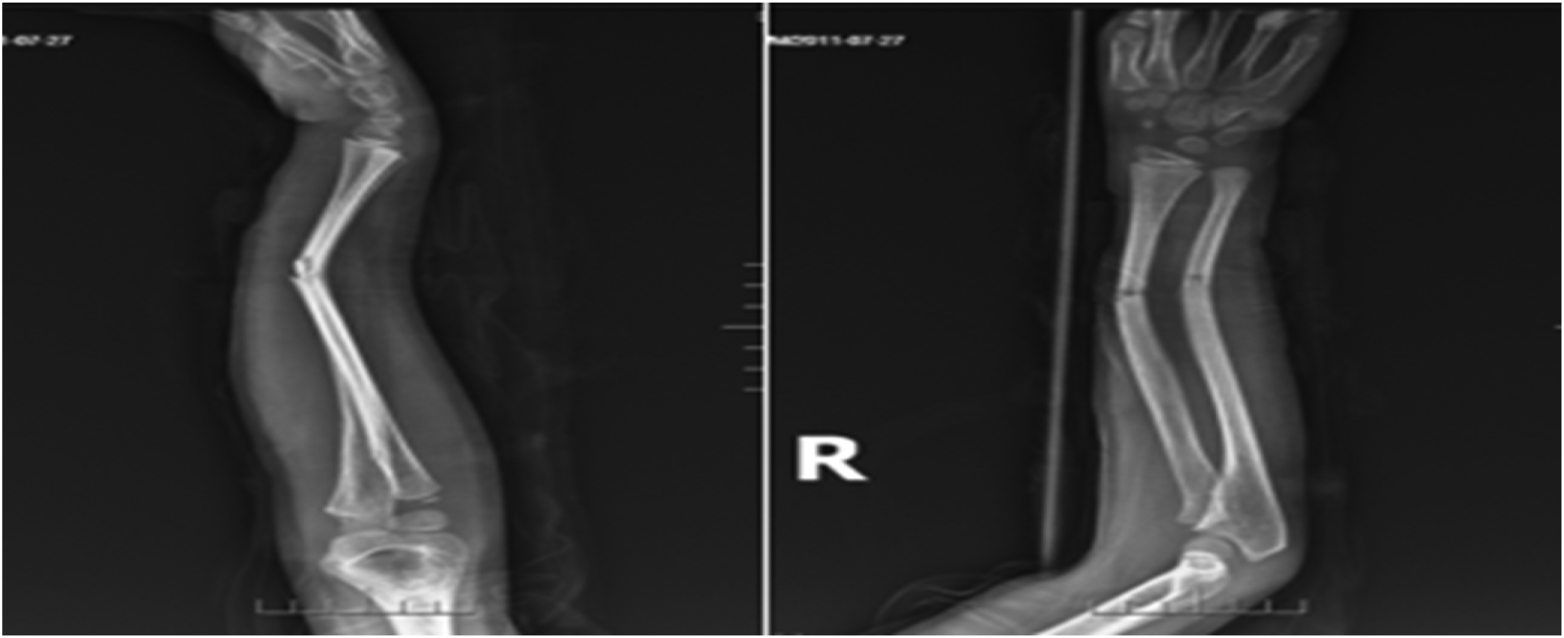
X-rays at the time of admission.
